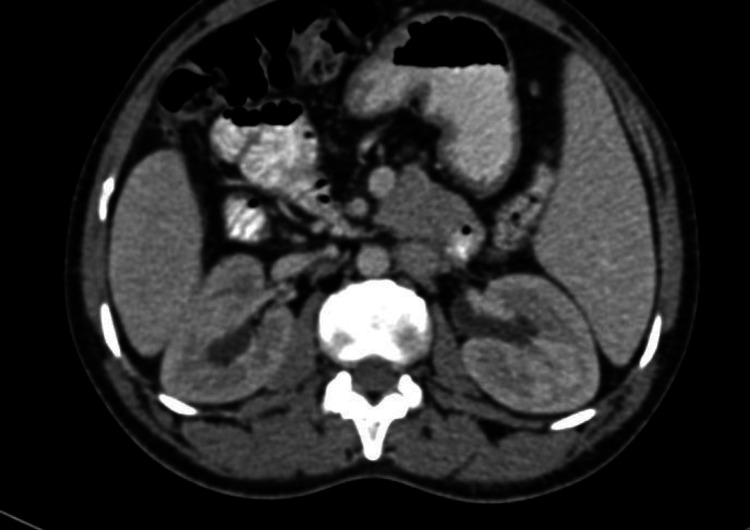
Axial enhanced CT showing mild periureteric fibrotic changes and mild hydronephrosis.
